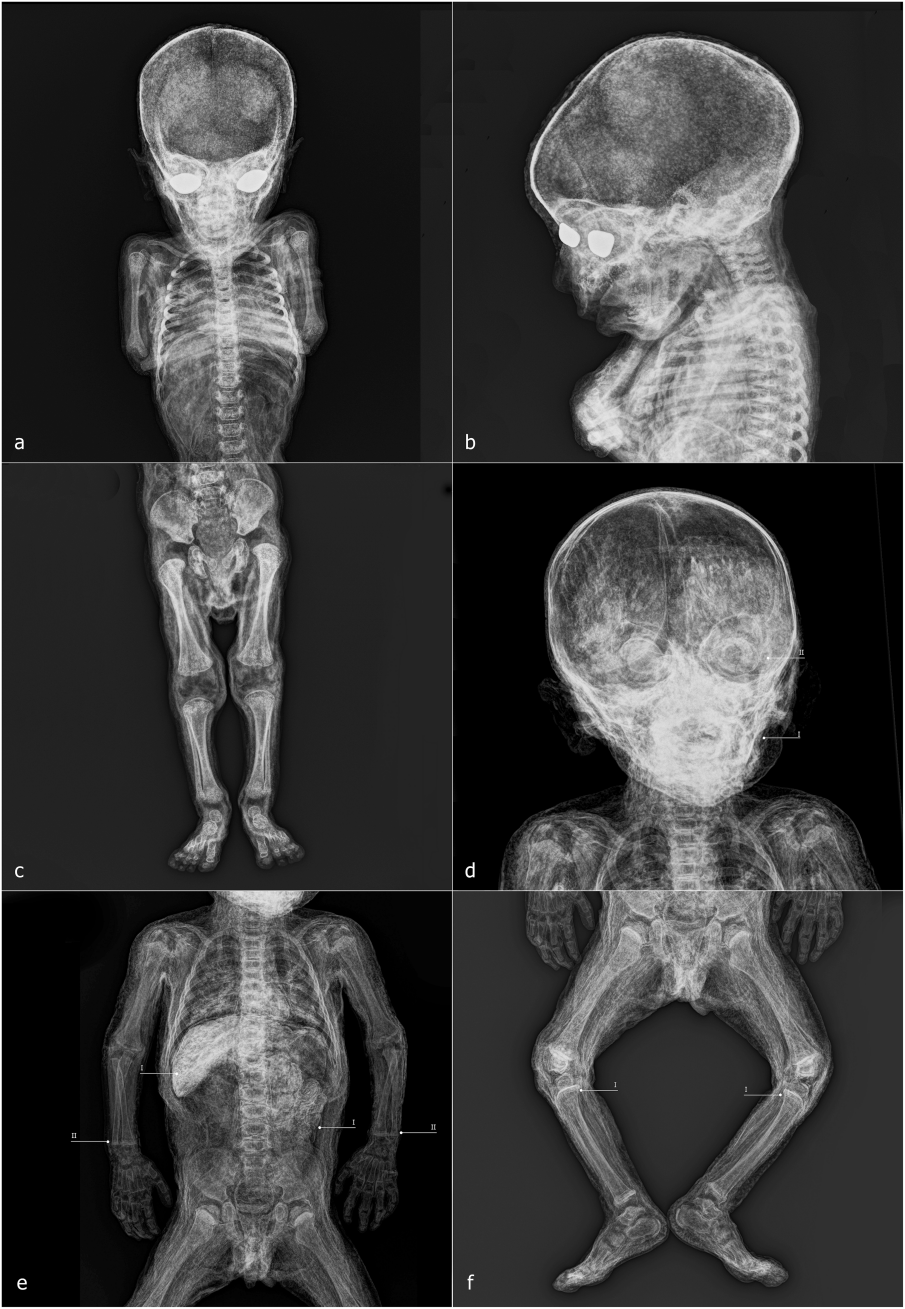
ID5—radiographic images: anteroposterior view of the skull and trunk (a); laterolateral view of the skull and shoulders (b); anteroposterior view of the lower limbs (c). ID6—radiographic images: anteroposterior view of the skull and shoulders (d); anteroposterior view of the trunk and upper limbs (e); anteroposterior view of the lower limbs (f).
